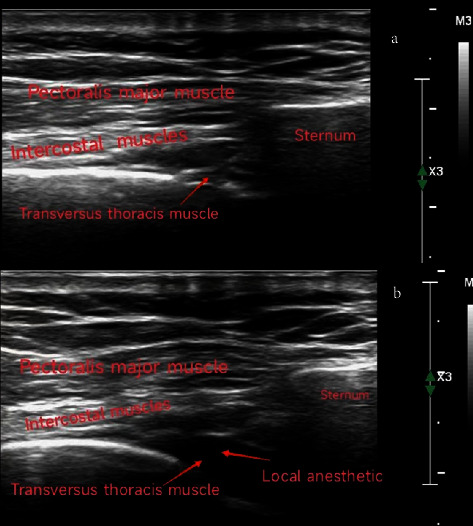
The ultrasound images in the TTMPB. (a) Ultrasound image before TTMPB; (b) ultrasound image after TTMPB. TTMPB: transversus thoracis muscle plane block.
